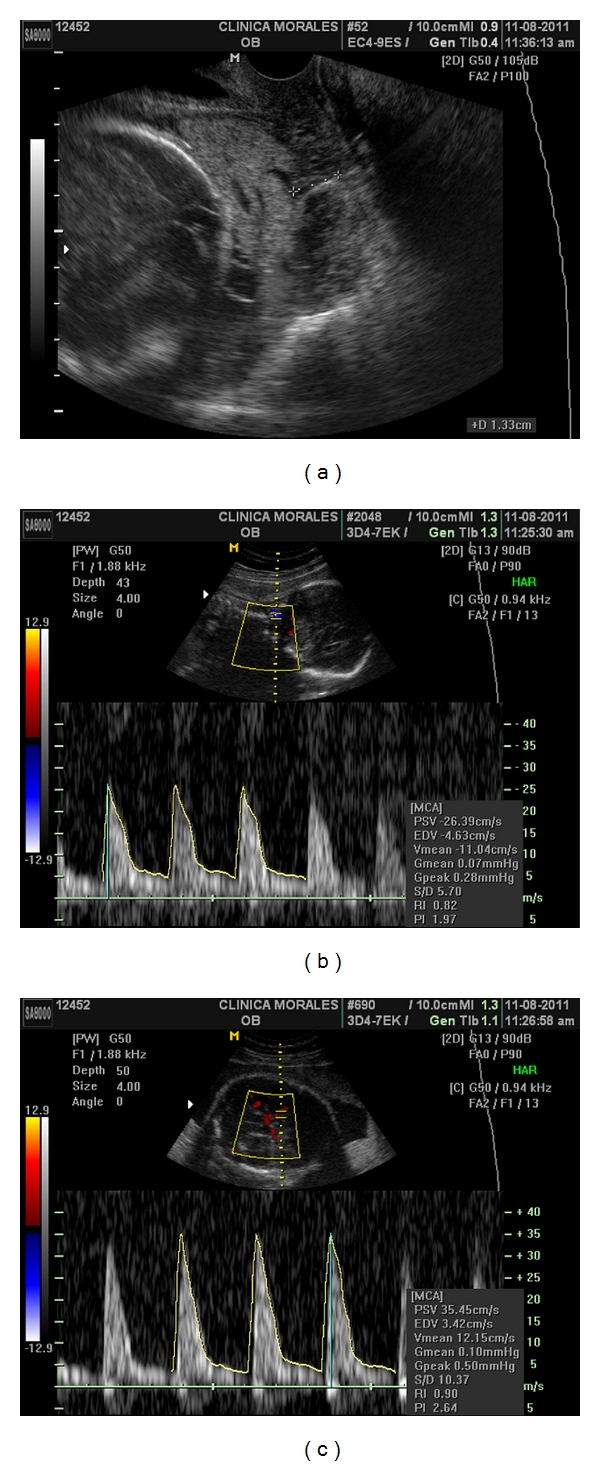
Week 28. Transvaginal colour Doppler ultrasound. The aberrant cotyledon is clearly seen covering the internal os but the cervix has become longer (13 mm) (a). There is a normalization of the VA and MCA impedance (RI 0.82/PI 1.97, RI 0.90/PI 2.64) (b, c).
